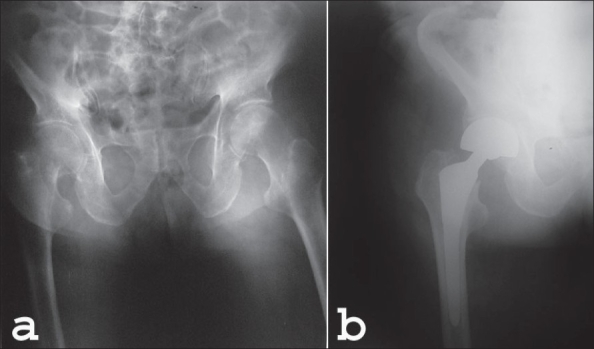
(a) Preoperative pelvis radiograph of a 84 year-old female with a right femoral neck fracture. (b) Followup X-ray of the same patient at 3 months. The initial filling and fitting of the medullary canal of the femur is good enough on the early postoperative radiograph
